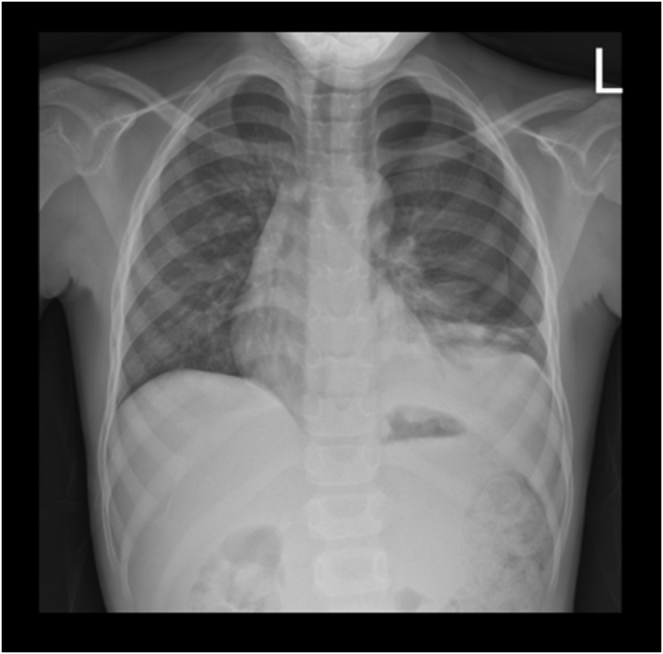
A control chest X-ray done after surgical repair of the diaphragmatic defect, showing no bowel loops in the left hemithorax.
